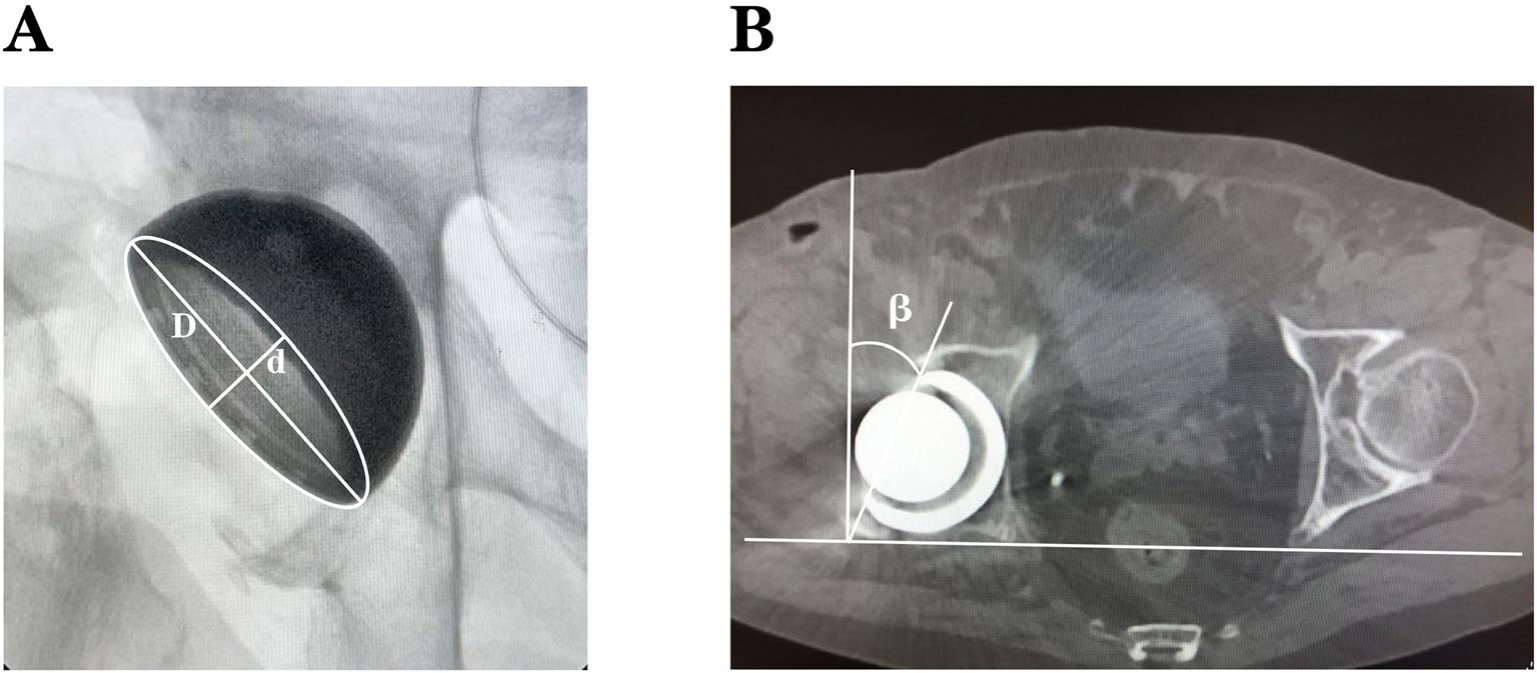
Measurement of the anteversion by the Lewinnek method (A) and CT scan (B). d: minor axis; D: major axis; β: anteversion.
